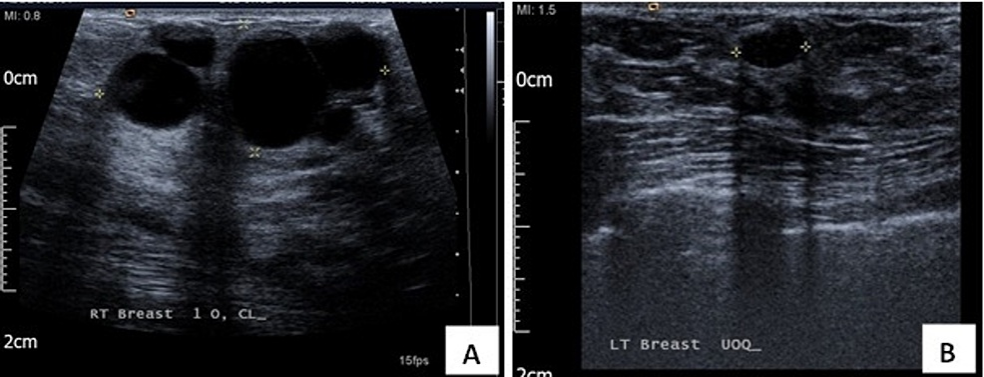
Ultrasound images. (A) Right breast: the entire breast displays an area of irregular abnormality. (B) Left breast: a 7 mm indeterminate lesion in the UOQ area. UOQ: upper outer quadrant
